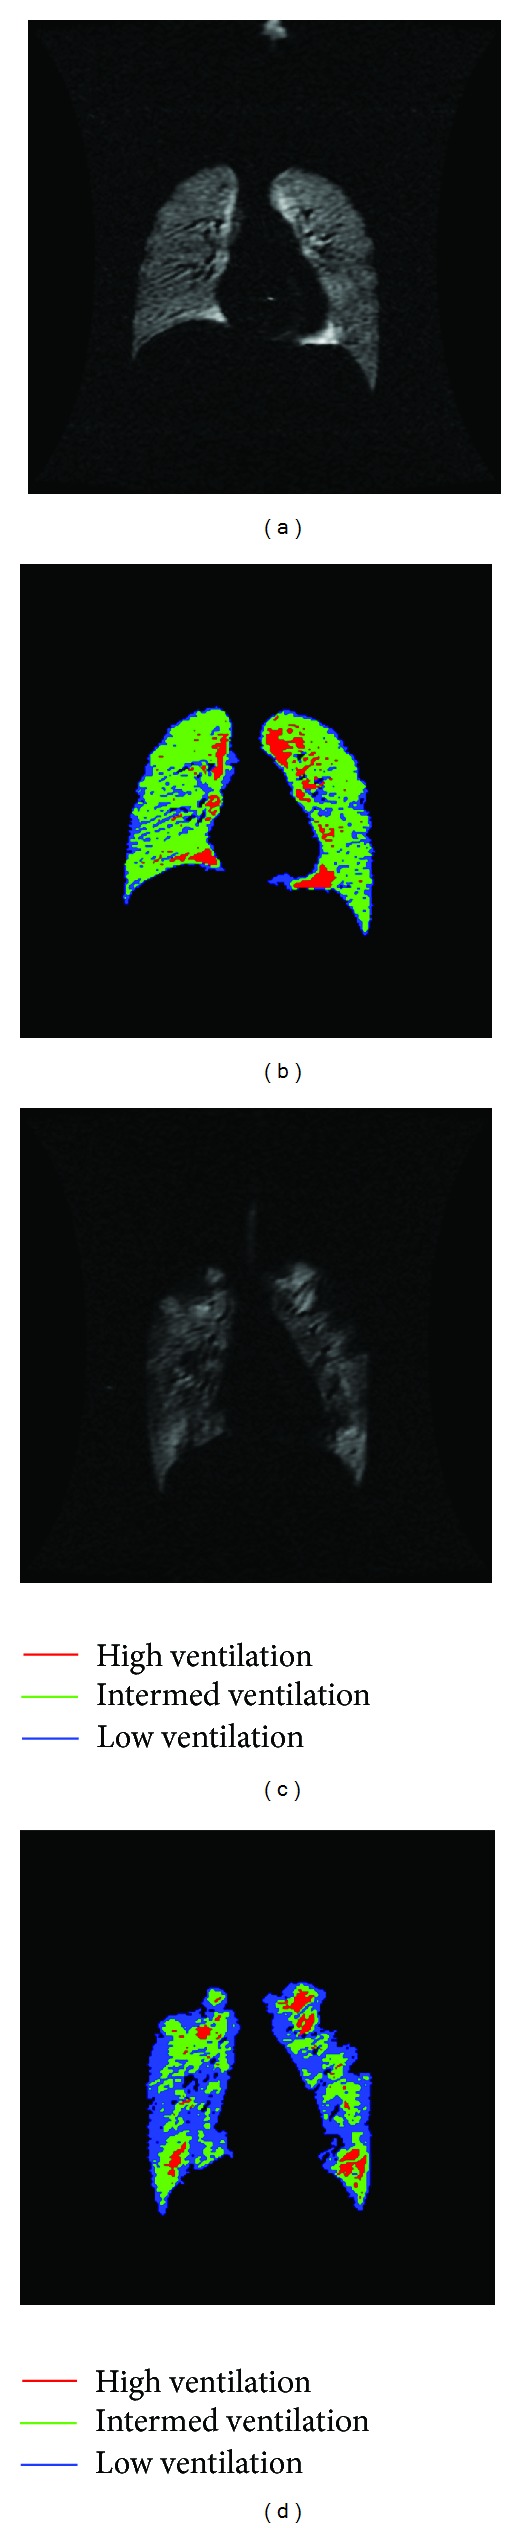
Segmented lung volumes for a healthy and an asthmatic subject. Panels (a) and (c) show a slice of the HP ^3^He MRI for a healthy and an asthmatic subject, respectively. The corresponding segmented images are divided into clusters of high, intermediate, and low ventilation as shown in panels (b) and (d). Note the increased predominance in pockets of hypointense areas indicating low ventilation in the asthmatic subject as compared to the healthy subject.
